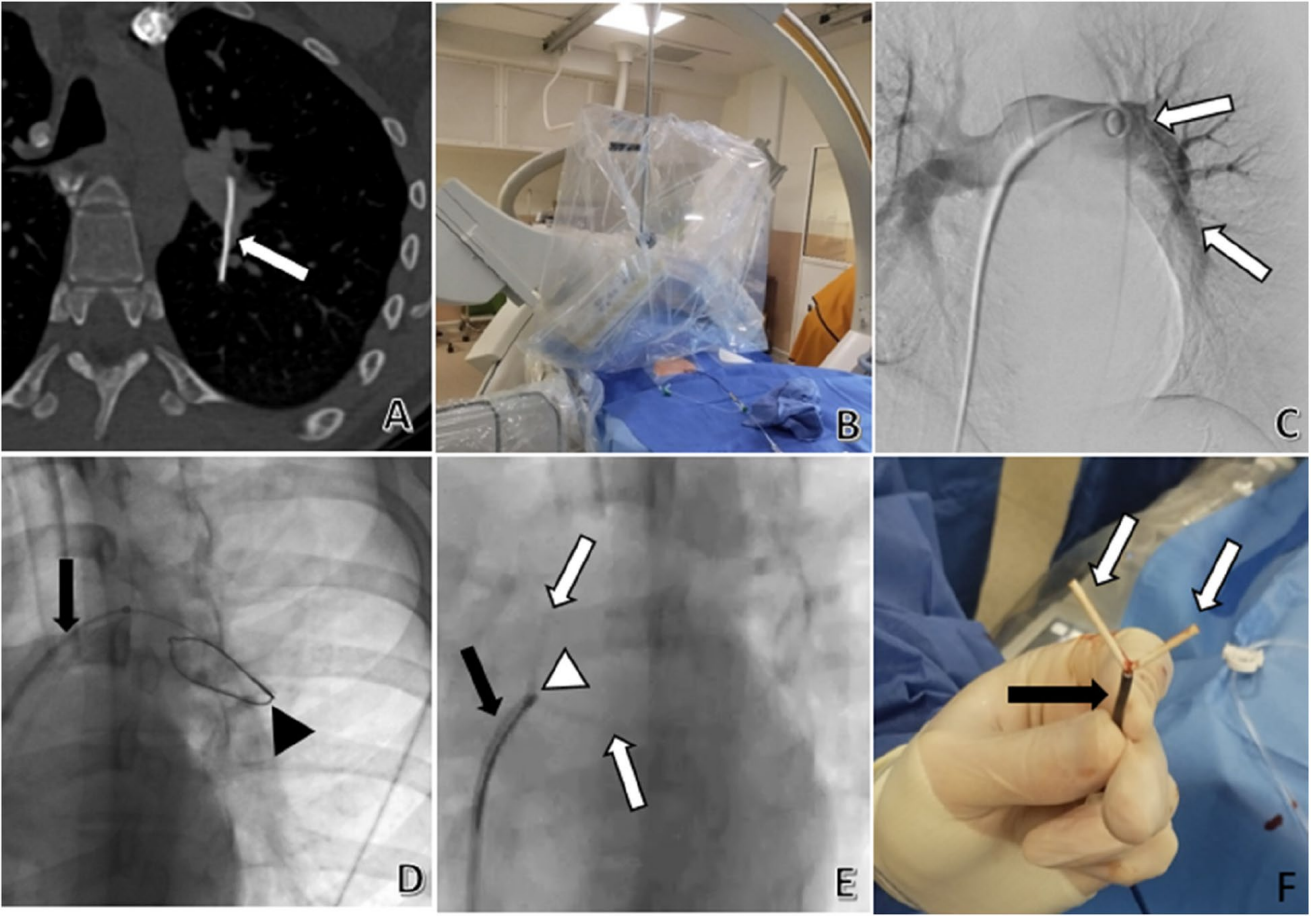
Endovascular retrieval of a contraceptive implant in the pulmonary artery. A A CT scan in the axial section showing a contraceptive device partially located in a left lower lobe segmental artery and the left pulmonary artery (white arrow). B The patient was placed in a dorsal decubitus position in a biplane interventional room, using both left and right anterior obliquities. C After a right femoral approach, an angiogram using a 5F pigtail showed the contraceptive device (white arrows), with no visible thrombosis. D A 8F introducer was placed into the left pulmonary artery (black arrow), allowing insertion of a 6F sheath and a loop snare (black arrowhead). E After pulling the contraceptive device (white arrows) with the loop snare (white arrowhead), it was then locked in front of the sheath and the introducer (black arrow). F After removing the entire introducer, the contraceptive device (white arrows) was trapped by the loop snare (black arrow)
